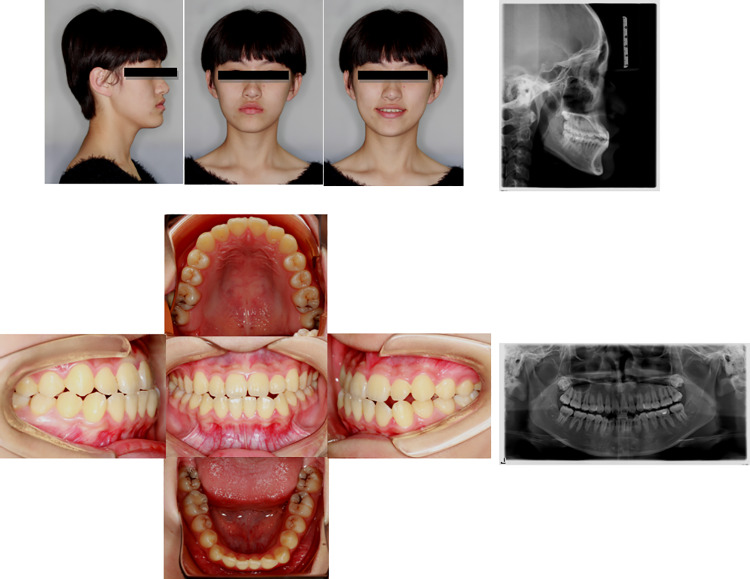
Pretreatment facial, intraoral photographs, and radiographs of the case with mandibular third molar extraction. These showed that the anterior mandibular process and bilateral molar relationships were mesial relationships with crossbite.
